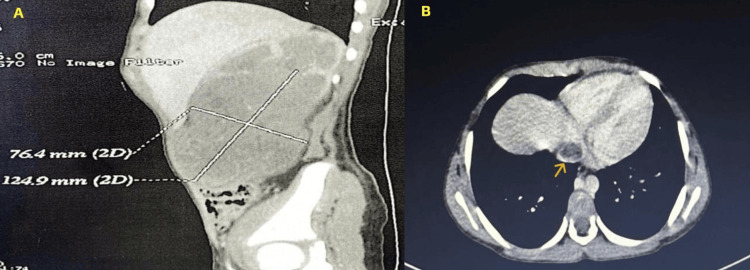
Thoraco-abdominopelvic computed tomography scan showing a nephroblastoma complicated by an inferior vena cava thrombus extending into the right atrium. A: Sagittal section of an abdominopelvic computed tomography scan showing a tissue mass occupying the lower two-thirds of the right kidney, consistent with nephroblastoma. B: Axial contrast-enhanced chest computed tomography scan showing a hypodense intraluminal material consistent with a thrombus in the inferior vena cava (arrow), extending up to its junction with the right atrium.
